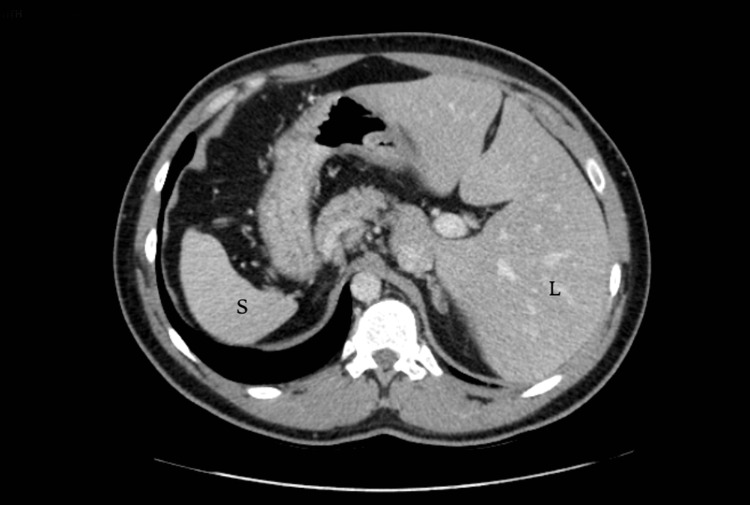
An axial view of an abdominopelvic CT scan showing a reversed location of the abdominal organs with the liver (L) positioned in the left side and the spleen (S) being in the right side
